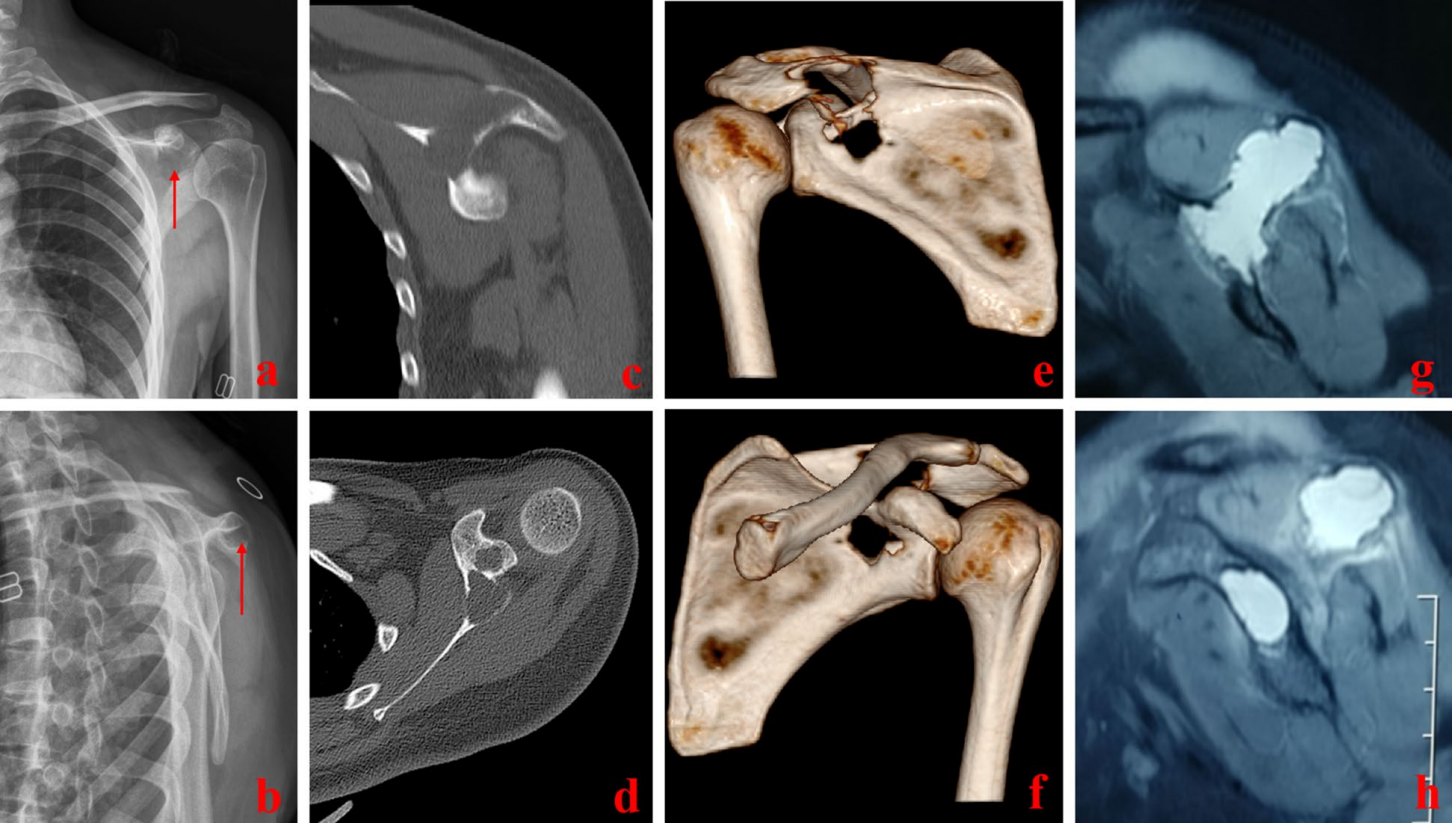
a and b are DR anteroposterior and lateral radiographs. Low density shadow can be seen in the scapular area with a clear boundary and a sclerosing zone on part of the edge. c and d show irregular and cystic bone destruction of CT, scapular spine and scapular neck in cross-sectional and sagittal position, respectively, and the bone cortex is punctured before and after perforation. e and f show three-dimensional reconstruction of the CT scapula, showing severe destruction of the scapula, especially the scapular bone, and penetrating the anterior and posterior bone cortex. g and h are T2 images of MRI, showing patchy abnormally high signal and lobulated images
